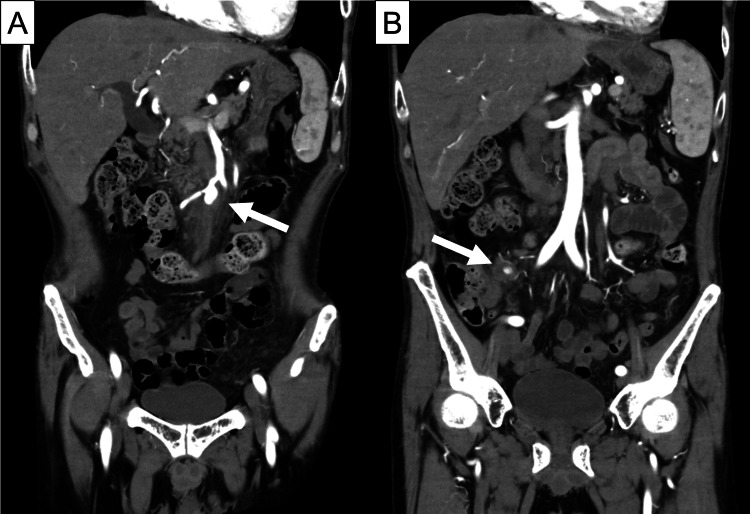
Abdominal contrast-enhanced computed tomography (CT) on day 0. Aneurysms of the ileal artery (A, arrow) and ileocolic artery (B, arrow).
